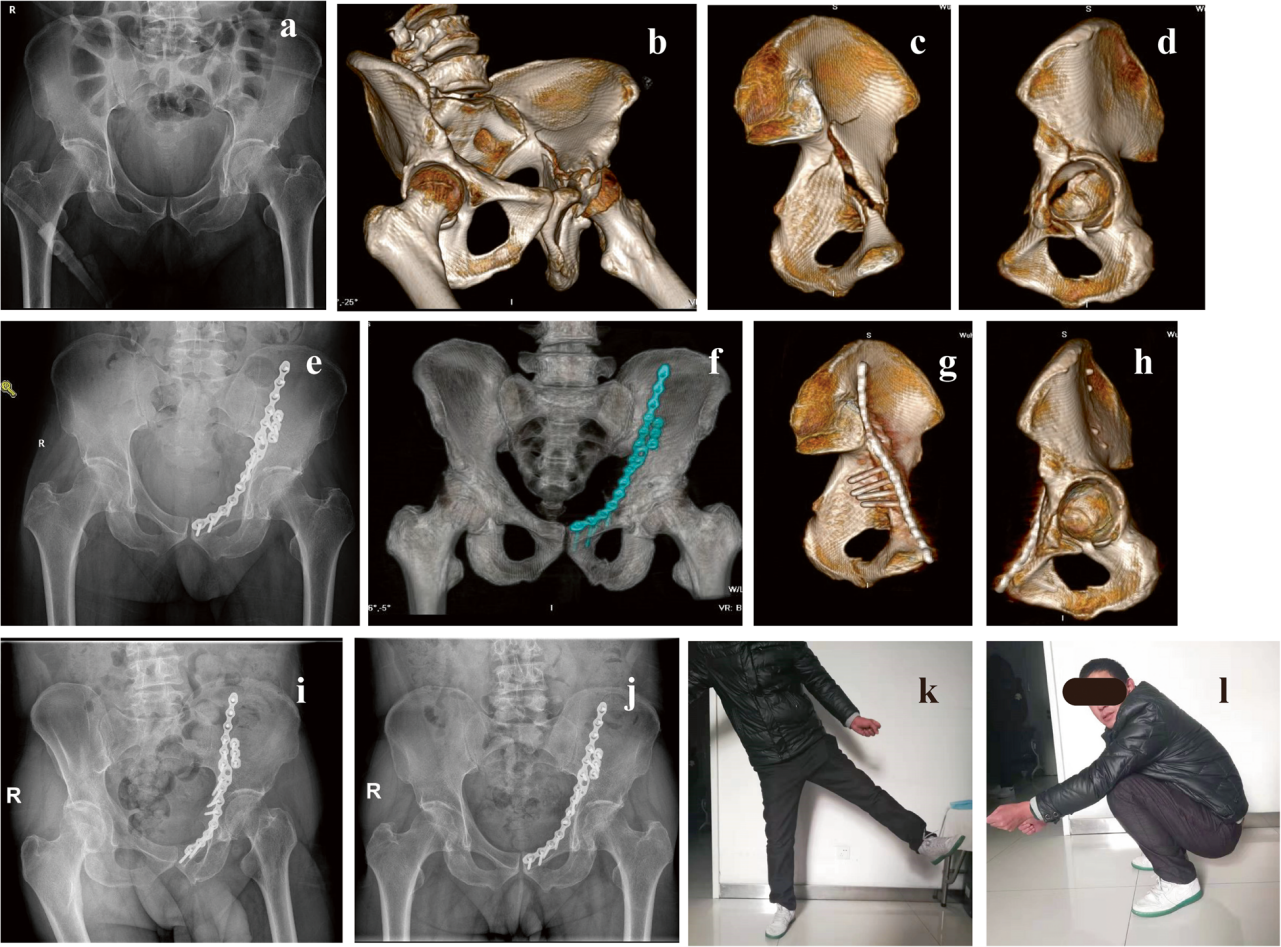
The second typical case showing the use of the standardized titanium plate for a complex acetabular fracture. A 56-year-old man presented with anterior column and posterior hemitransverse fracture of the left acetabulum following a traffic accident. Preoperative AP (a) and 3D CT reconstruction (b–d) of the pelvis have confirmed the fracture pattern. On the 9th day after the injury, fixation was performed through the ilioinguinal approach using the type I standardized titanium plate with 4 quadrilateral screws. Postoperative AP (e) and 3D CT reconstruction (f–h) showing Matta’s X-ray evaluation was scored as excellent. The AP (i) and Judet views (j) after 1 year showing a good radiological result and no evidence of osteoarthritic change. The modified Merle d’Aubigné evaluation was scored as excellent and the hip function of abduction (k) and flexion (l) was satisfied
